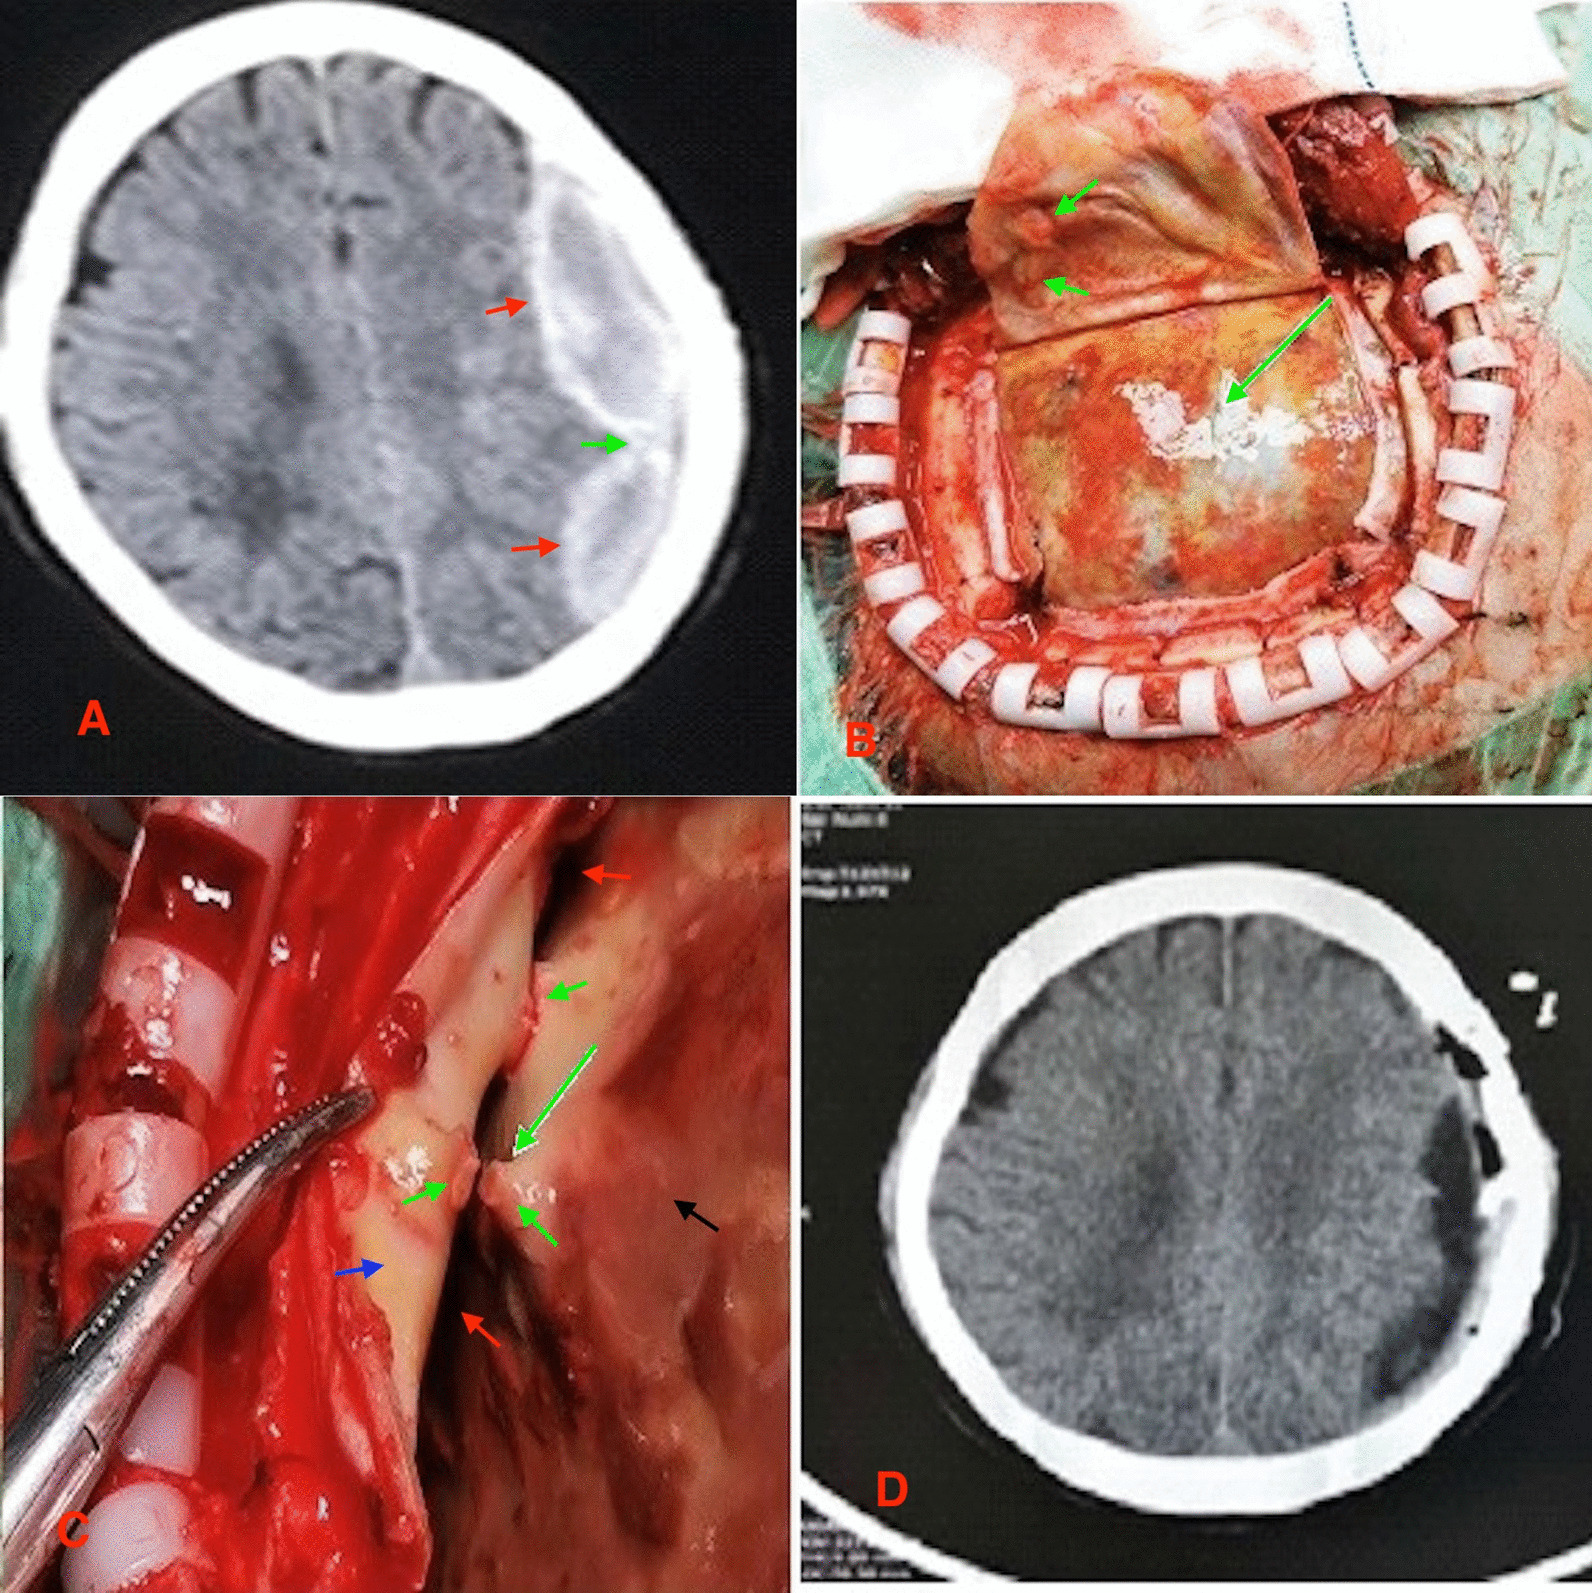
Preoperative CT scan, intraoperative images and postoperative CT scan on one of the patients without completely liquefied hematoma and hematoma cavity separation who underwent craniotomy. A preoperative CT image: *Red arrow* = None liquefied hematoma; *Green arrow* = thickened membranes connecting/separating the dura and the thickened arachnoid/pia matters. B, C Intraoperative images showing neomembrane, hematoma cavity separation as well as thickened membranes connecting/separating the dura and the thickened arachnoid/pia matters. B: *Green* arrows = thickened dura separators. C: *Green arrows* = thickened membrane connecting/separating the dura and the thickened arachnoid/pia matters, *Red arrows* = hematoma cavity separation, *Black arrow* = neomembrane and *Blue arrow* = dura. D Postoperative CT scan showing evaluation of the hematoma and separation of the thickened membranes connecting/separating the dura and the thickened arachnoid/pia matters
